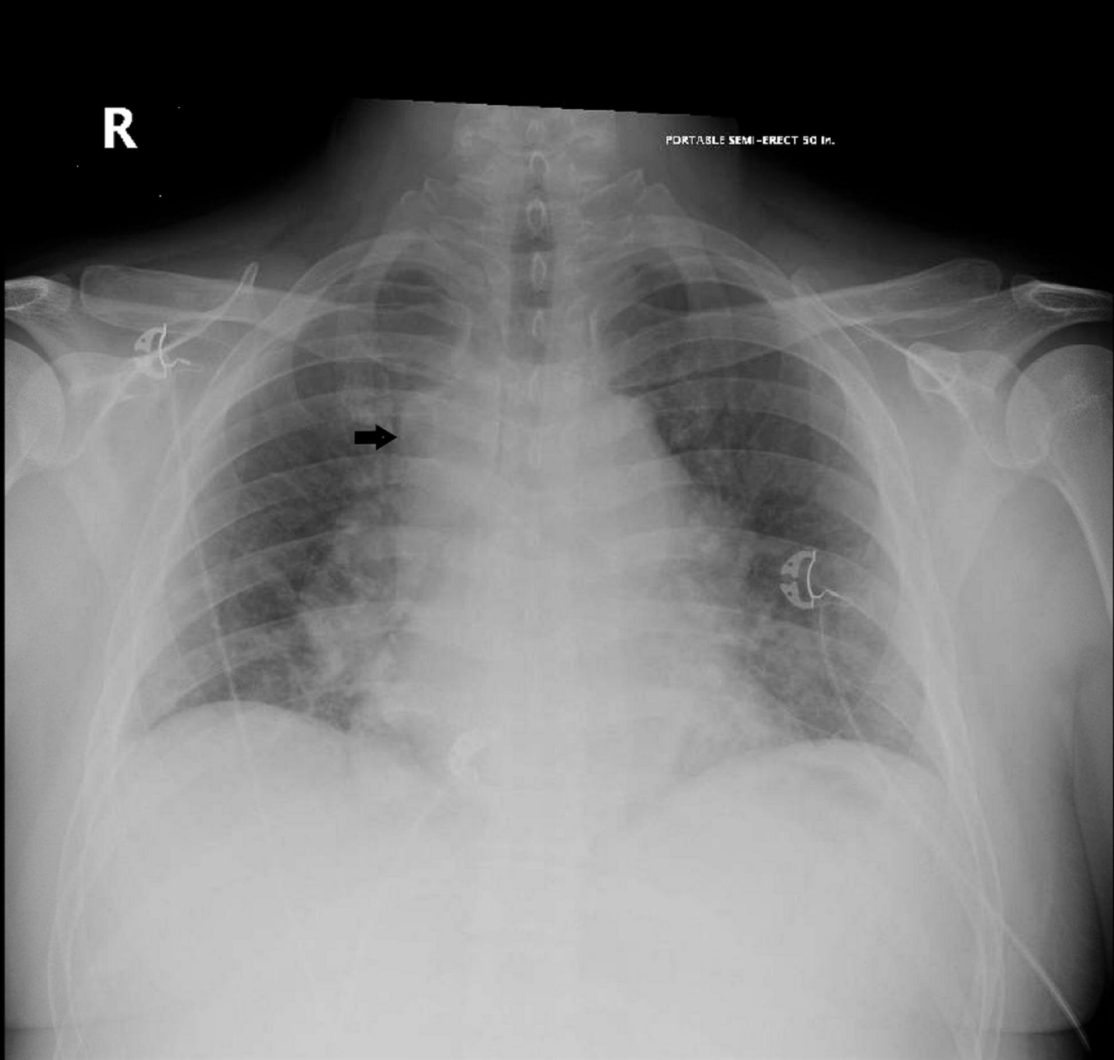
Chest x-ray with ill-defined opacity overlying the mediastinum (black arrow)
